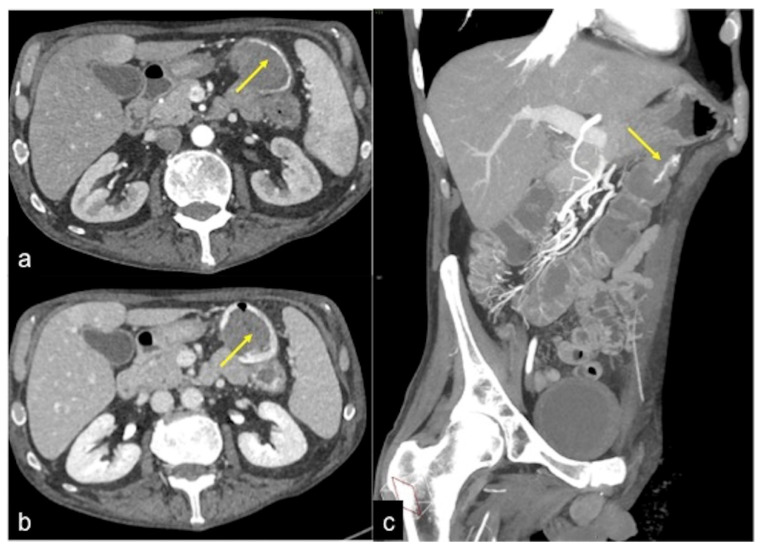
Different radiologic pathways of bleeding. CTA multiphasic study in arterial (a) and venous (b) phases reveals intestinal bleeding with circular morphology (a,b, arrow) along the endoluminal wall of splenic flexure with enlargement and intensification in the venous phase. The MPR reconstruction of the arterial phase in the coronal-oblique plane (c) shows the whorled morphology of bleeding (arrow).
